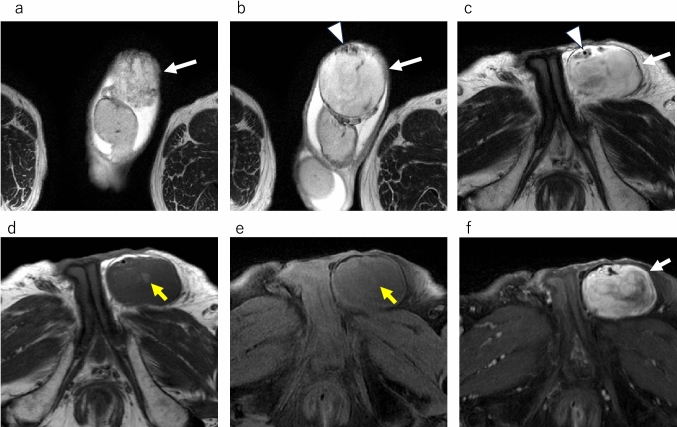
Liposarcoma in an 83-year-old male with the left scrotum swollen. a–c Axial T2-weighted MR images demonstrate hyperintense mass (white arrow) in the left scrotum. The mass is located in the left inguinal canal, and the mass compresses the spermatic cord (white arrowhead) anteriorly. d Axial T1-weighted MR image shows a small hyperintense area (yellow arrow) in the mass. e Axial fat-suppressed T1-weighted MR image shows hypointense area (yellow arrow) that is hyperintense on T1-weighted MR image (d, yellow arrow), suggesting that the tumor contains fat component. f Axial contrast-enhanced fat-suppressed T1-weighted MR image shows heterogeneous enhancement of the mass (white arrow)
